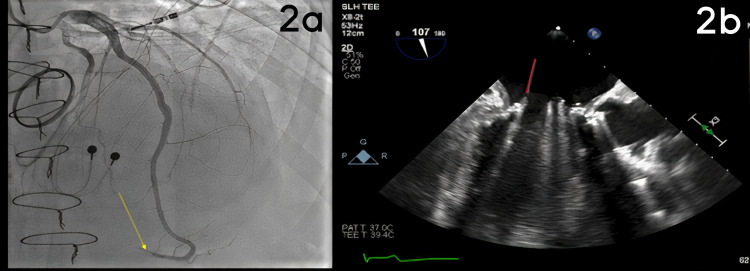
(a) Yellow arrow points to distal LAD thrombus in LAO cranial view. (b) Red arrow points to vegetation on the mitral valve in TEE. LAD: Left Anterior Descending Artery; LAO: Left Anterior Oblique; TEE: Transesophageal Echocardiogram
